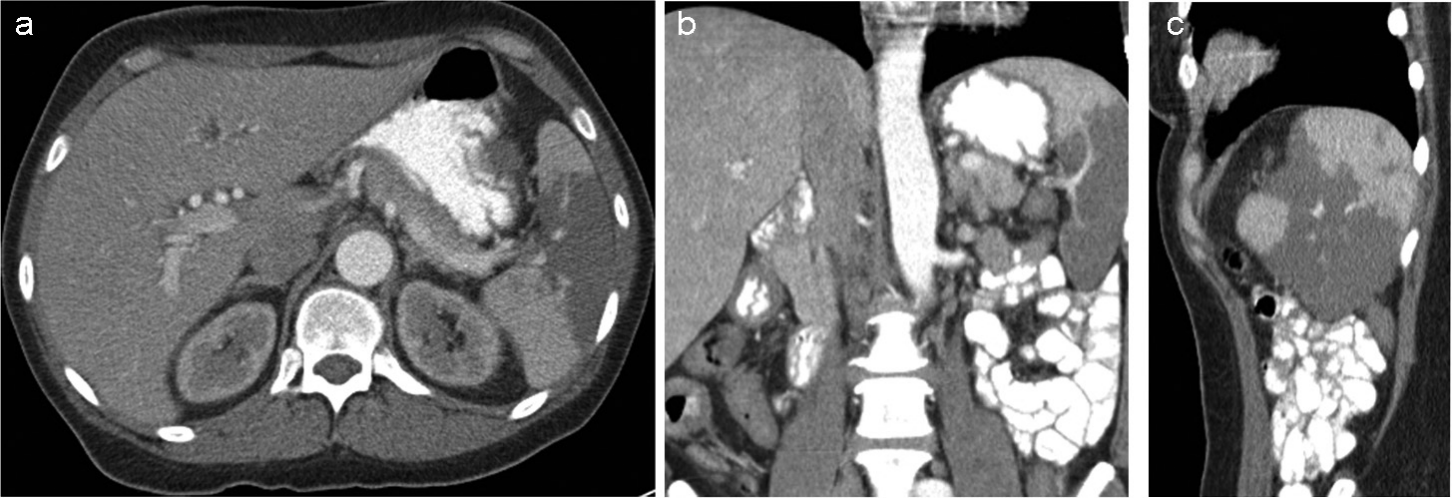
Three views (axial, coronal, and sagittal) from the contrast-enhanced computed tomography scan of the abdomen demonstrate the large wedge-shaped region of decreased radiodensity within the spleen representing the area of infarction.
